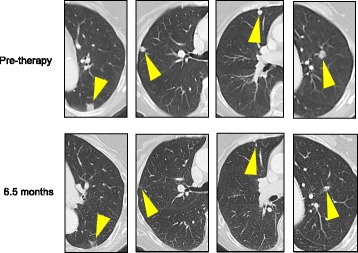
Partial response of metastatic basal cell carcinoma to pembrolizumab (anti-PD-1). Computed tomography (CT) scans performed pre-therapy (*top row*) and 6.5 months after initiating pembrolizumab (anti-PD-1, *bottom row*) demonstrate regression of basal cell carcinoma lung metastases. *Yellow arrowheads* indicate sites of metastases
